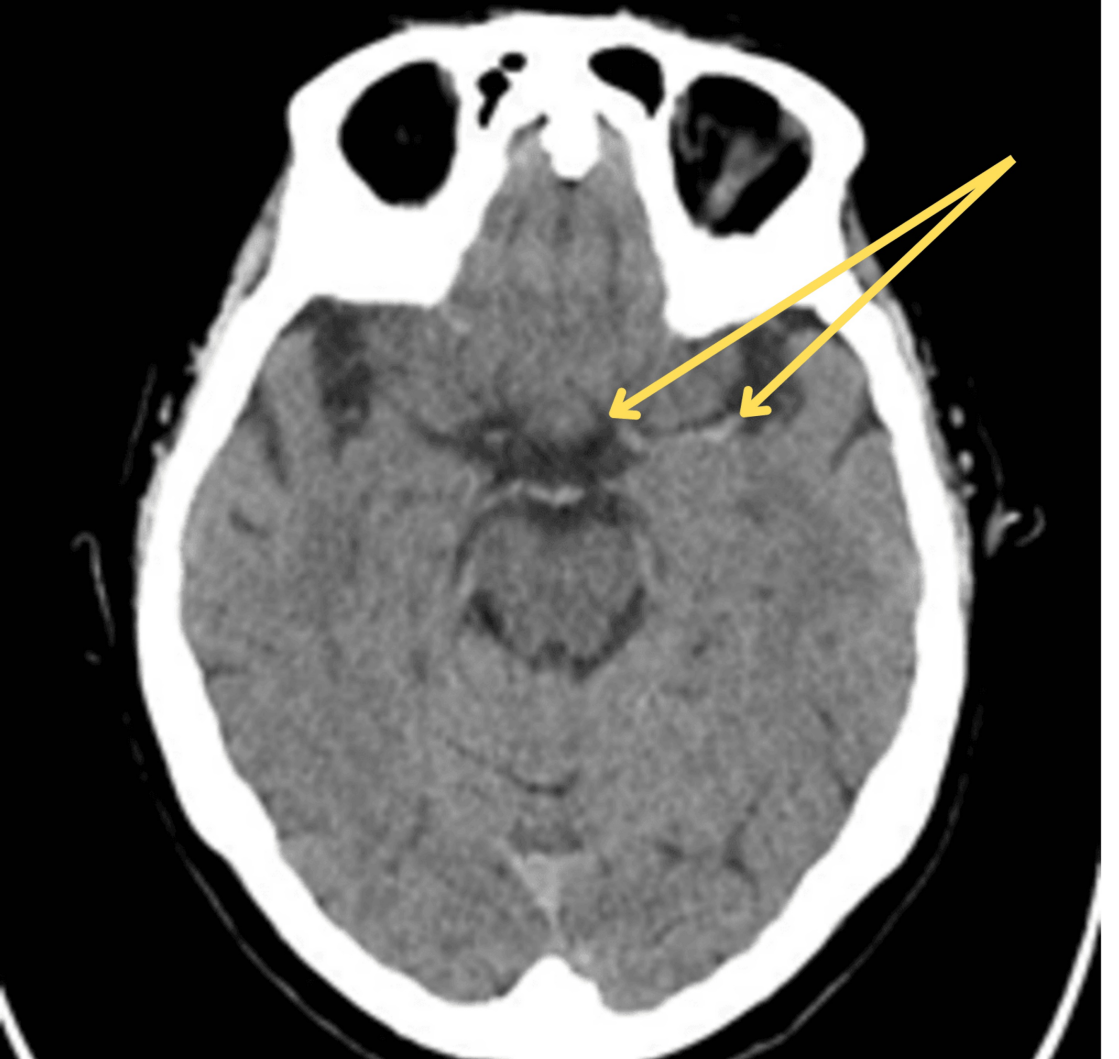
Axial section of non-contrast CT head showing hyperdense left middle cerebral artery
